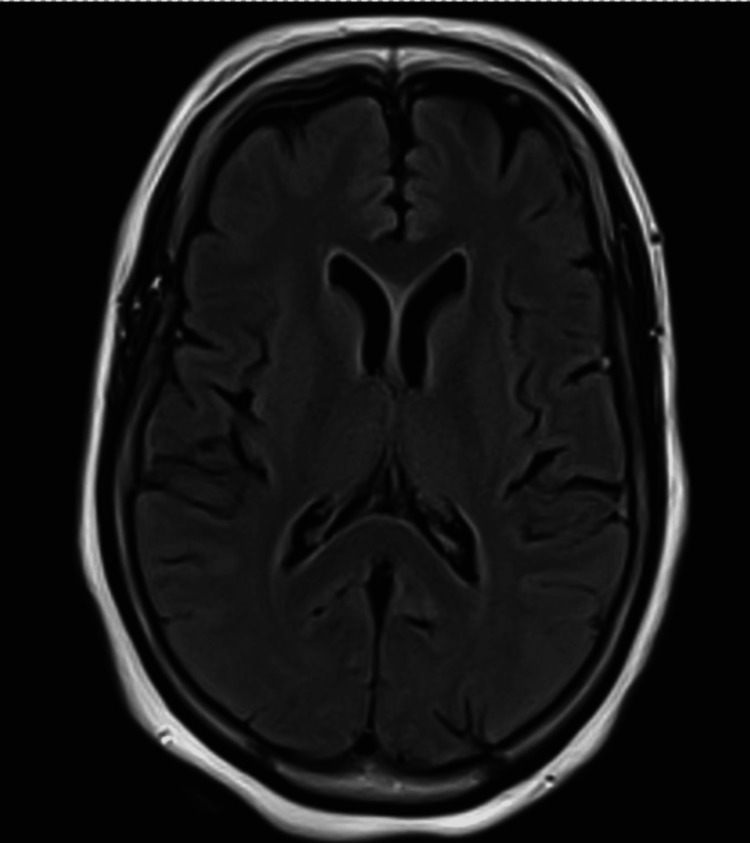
FLAIR image MRI brain, axial view, with no demonstrable significant findings. FLAIR: fluid-attenuated inversion recovery, MRI: magnetic resonance imaging
